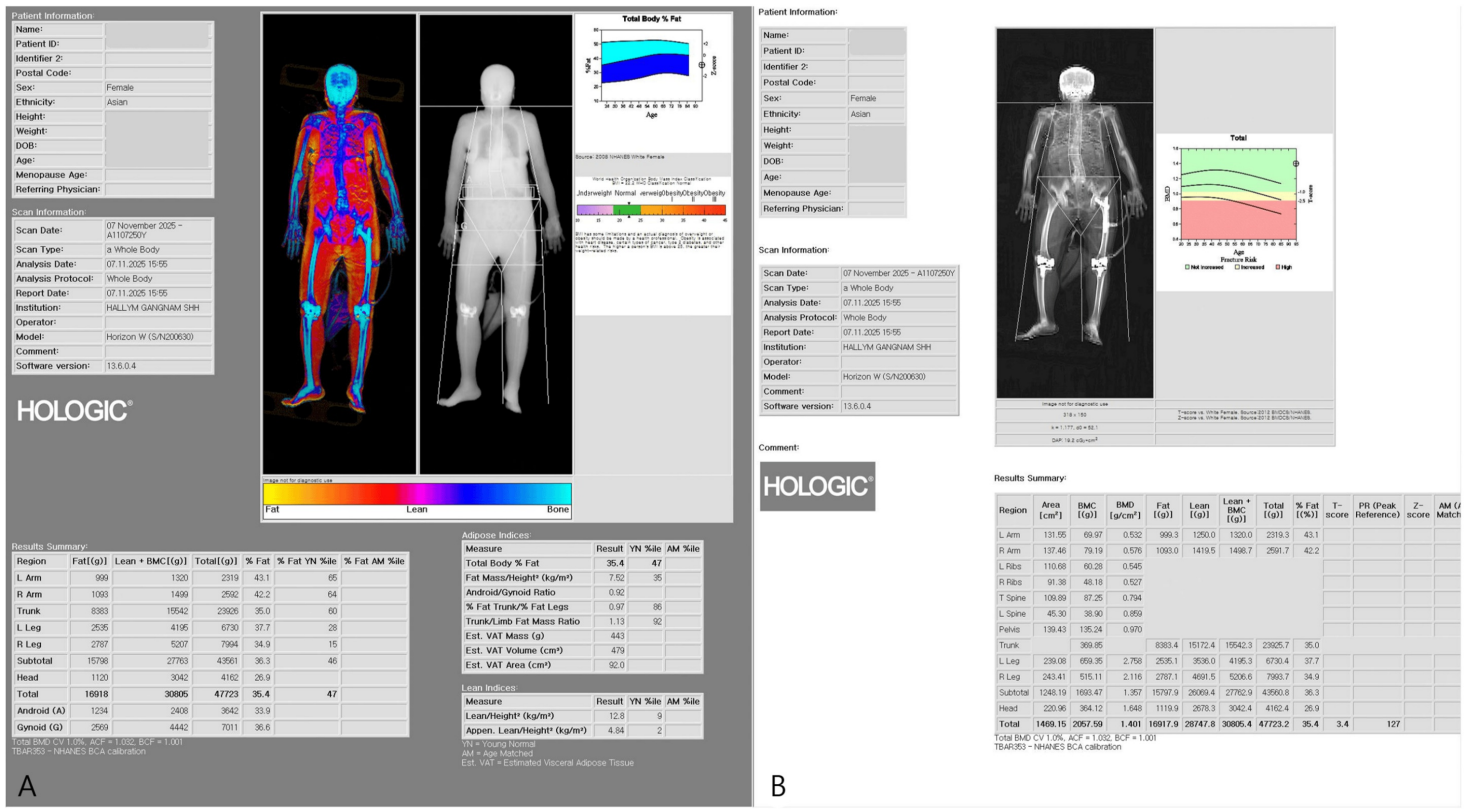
DXA-based body composition analysis (A) Global body composition indices, including ASMI, SMI, and body fat percentage. (B) Regional measurements of BMD, BMC, fat mass, lean mass, and LB for each body segment DXA: dual-energy X-ray absorptiometry; ASMI: appendicular skeletal muscle index; SMI: skeletal muscle index; BMD: bone mineral density; BMC: bone mineral content; LB: lean mass plus bone mineral content
